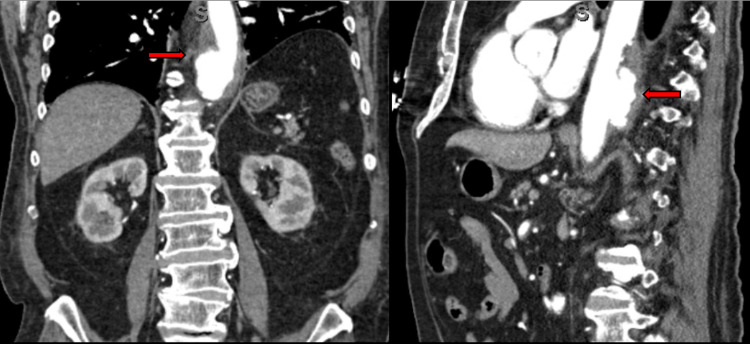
CT angiogram of the chest, abdomen, and pelvis revealed a distal descending aorta dilated aneurysm of 7.6 cm with a proximal fusiform aspect and the presence of a moderate plaque or thrombus along the posterior aspect of the aorta and displaced intimal flap (horizontal red arrows). Moderate atherosclerotic plaque with calcified and noncalcified plaque was present within the aortic arch and descending aorta.
